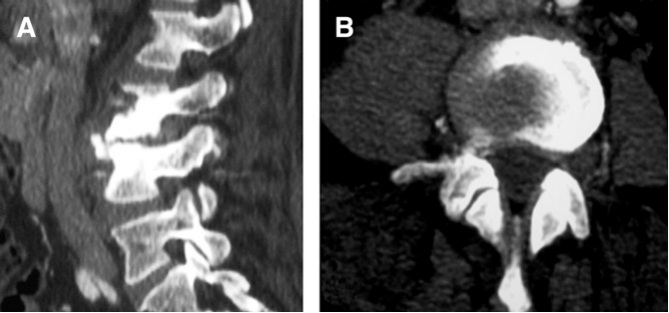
(A) CT scan of the spine showing erosions on the antero-superior angle of the vertebral body of L3, narrowing of the intervertebral disc space between L3 and L4, erosions on the end plates of these two vertebral bodies, and new bone formation. (B) Widening of the right iliopsoas muscle.
